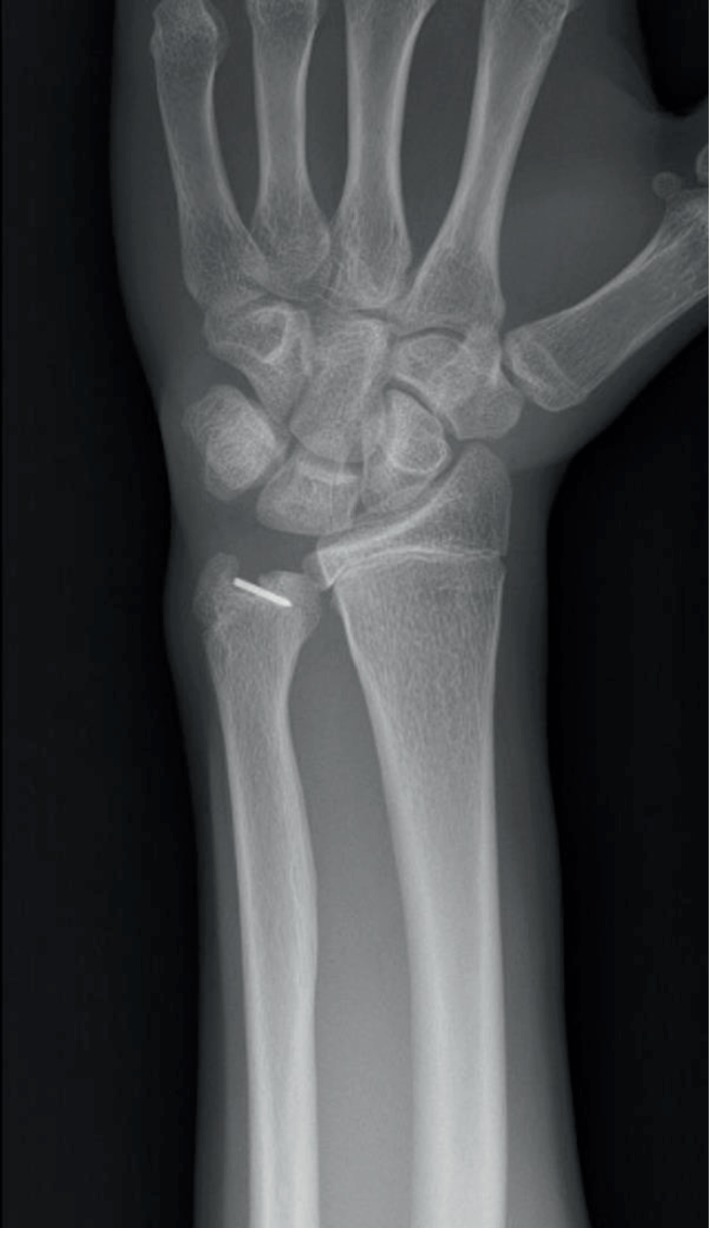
Anterioposterior and lateral X-ray 1 year after surgery shows premature physeal closure and mild negative ulnar variance.
